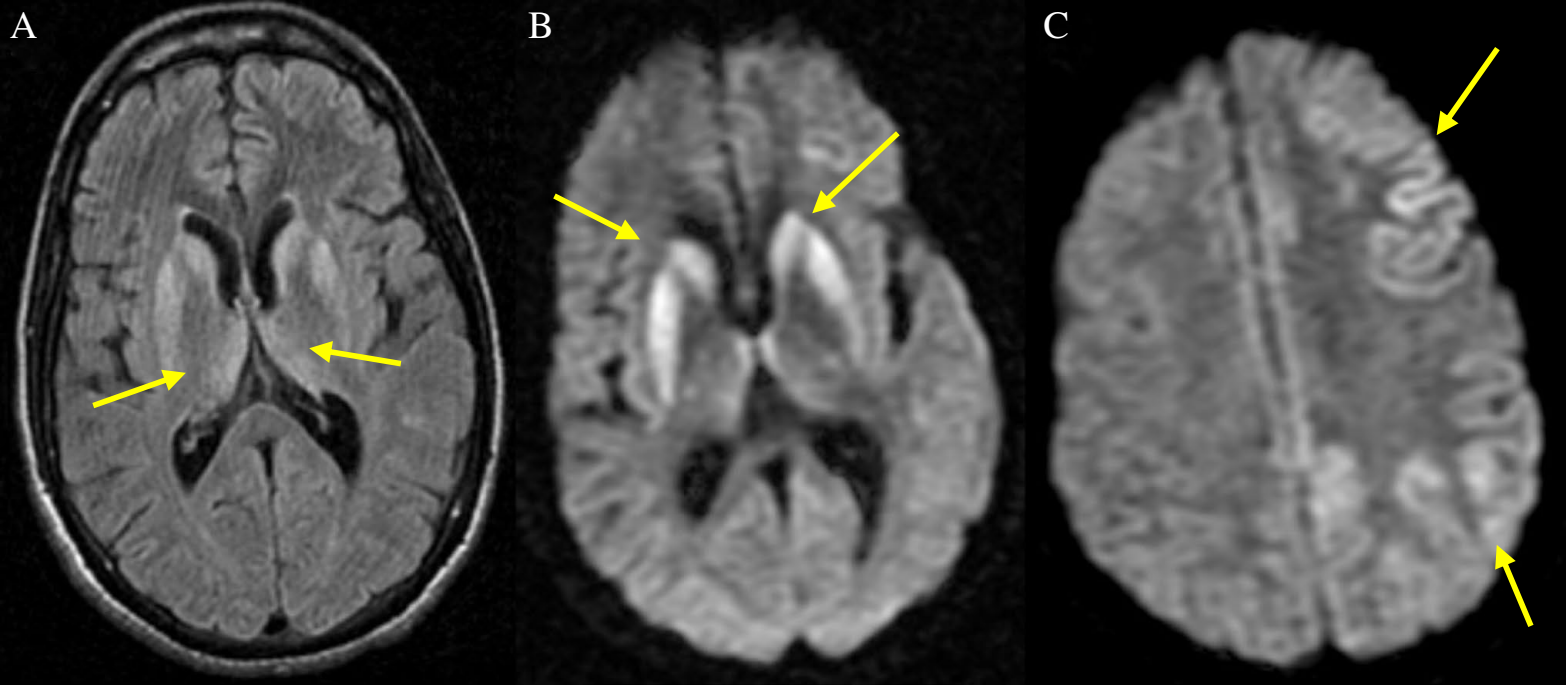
MRI in a confirmed case of vCJD with appearances more characteristic of sporadic CJD (A) Axial FLAIR showing mediodorsal and pulvinar high signal (arrows) of equal intensity to basal ganglia. (B) DWI showing more conspicuous high signal in basal ganglia (arrows) with less marked dorsomedial thalamic abnormalities and (C) associated left parietal and frontal cortical hyperintensity (arrows)
